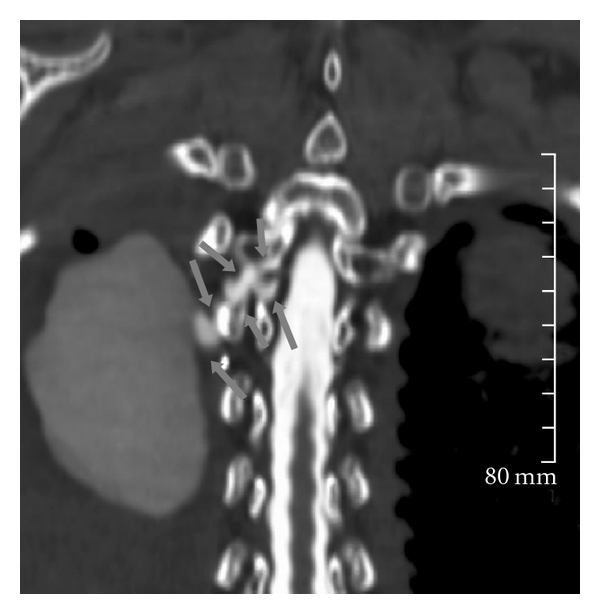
Coronal reformatted CT after contrast myelogram shows the subarachnoid pleural fistula (arrows) connecting the radio-opaque subarachnoid space with the large area of dilute contrast in the right pleural space.
